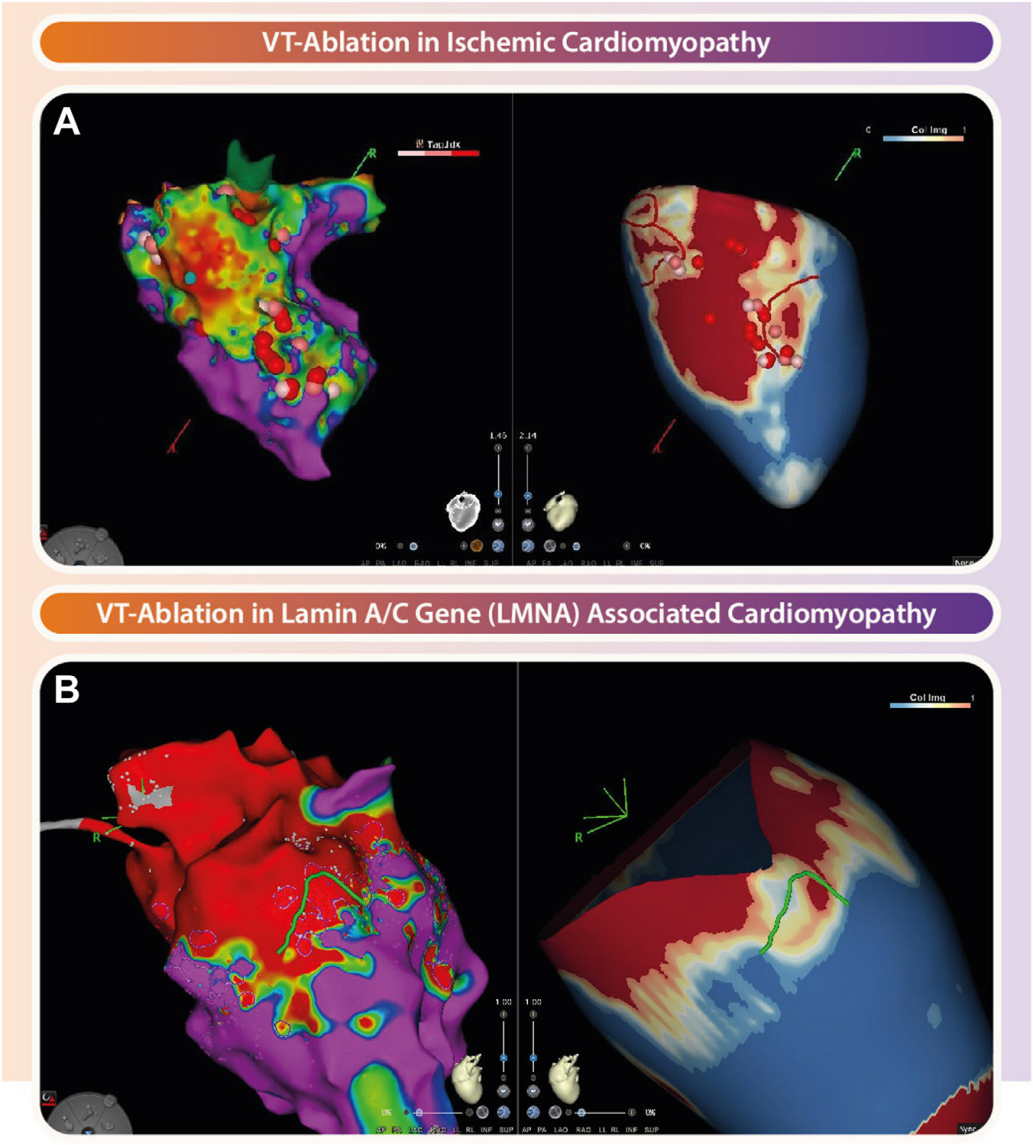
Role of magnetic resonance imaging–derived scarmaps in guiding ventricular tachycardia (VT) ablation. A: Electroanatomic map (EAM) (left) and cardiac magnetic resonance–derived scarmap (right) of a patient with ischemic cardiomyopathy where ablation of the conduction channel (CC) rendered the VT noninducible. B: Comparable overview with EAM (left) and scarmap (right) of a patient with nonischemic cardiomyopathy where activation mapping was not feasible due to hemodynamic instability during VT. Fourt different VT foci were identified using targeted mapping in regions with border zone and CCs. The CC, which traversed through a large midwall septal substrate, was ablated, resulting in noninducibility of the clinical VT.
